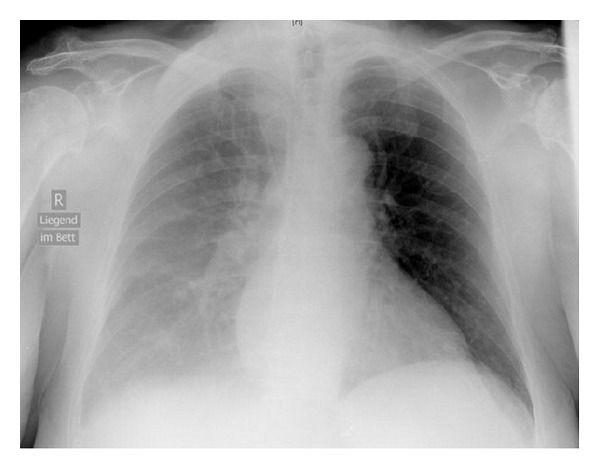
Chest X-ray anteroposterior after puncture (lying).
